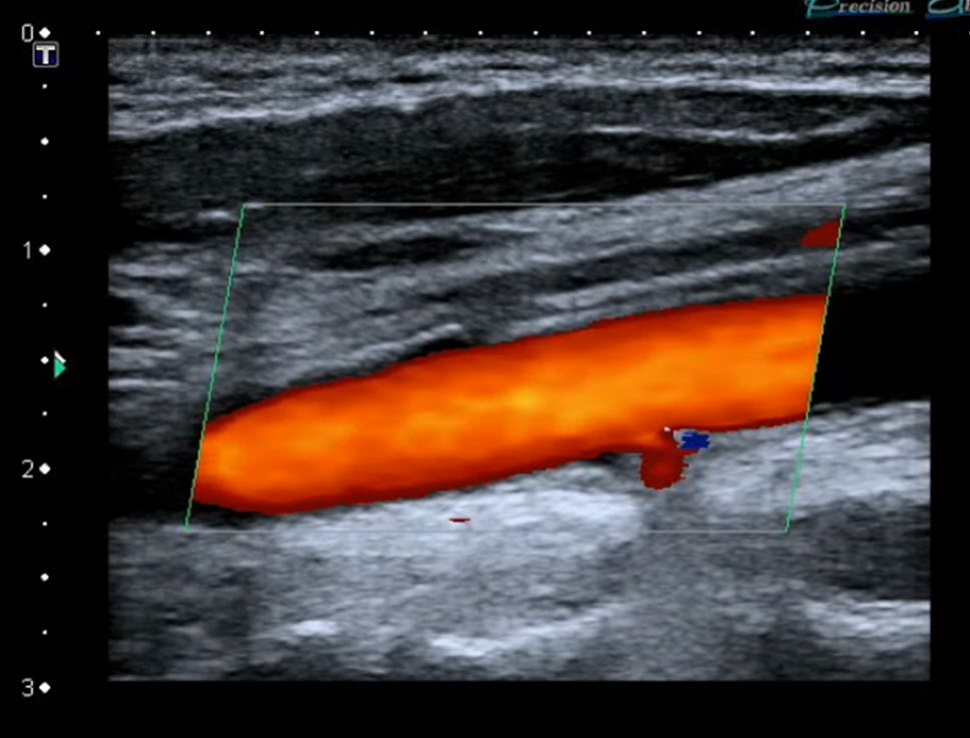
Color Doppler Imaging of carotid demonstrating turbulent flow in the posterior wall pseudoaneyrysm
